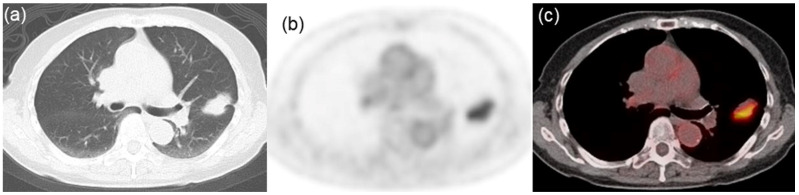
Representative images from an 81-year-old woman with lung adenocarcinoma characterized by negative PD-L1 expression. (a) CT image indicating a lung mass in the left upper lobe. (b) FDG PET and (c) PET/CT fusion images exhibiting high tracer uptake within the tumor (SUVmax = 5.23, LGRE = 0.0107, SRLGE = 0.0099, LRHGE = 158, LGZE = 0.0120, HGZE = 107, and SZLGE = 0.0055).
